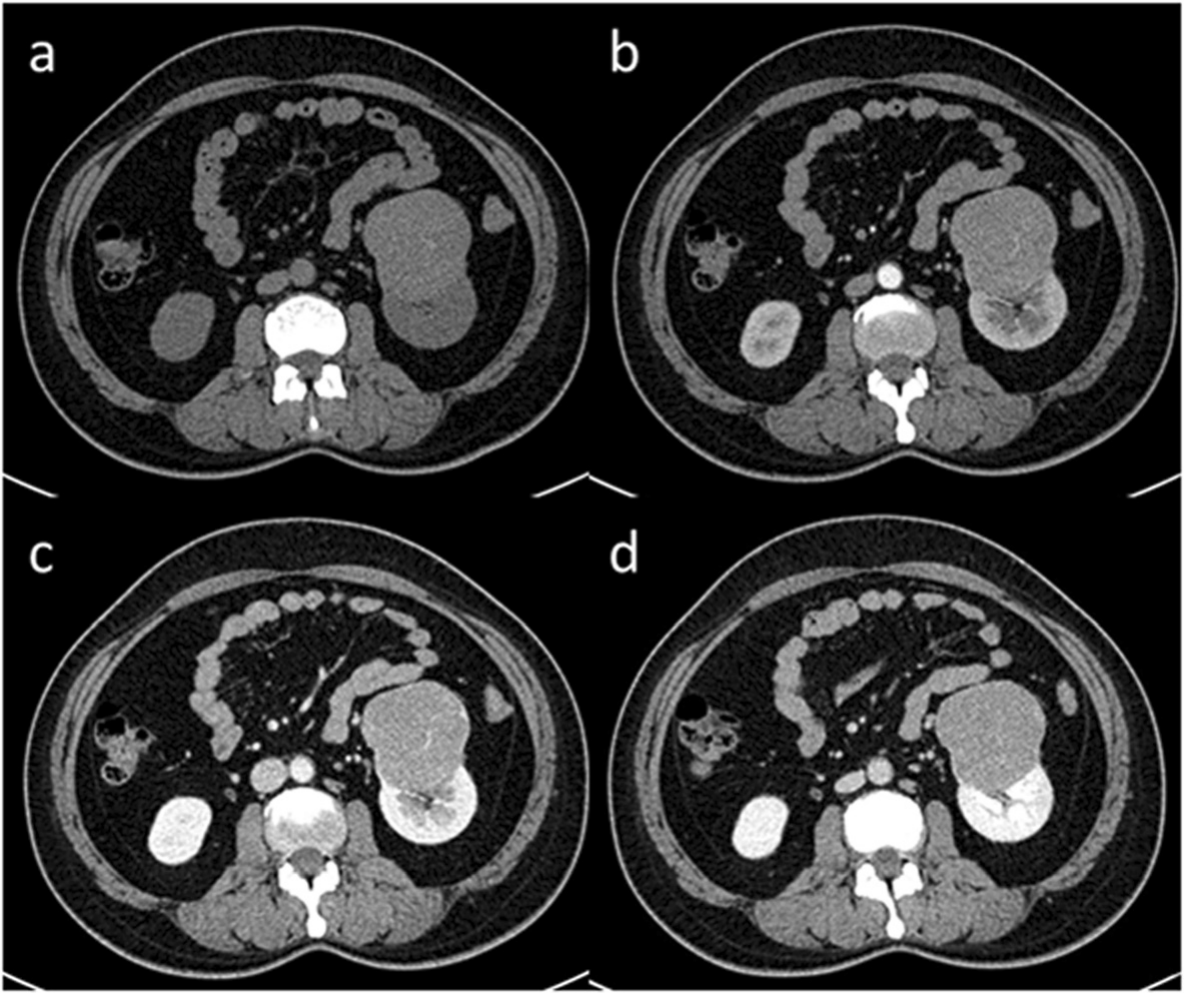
A 45-year-old woman with E-AML. The patient had no clinical symptoms. A renal mass was found during physical examination. Non-contrast axial CT image (a) demonstrates a hyper-density lesion with lobulated appearance in the lower renal pole of the left kidney. Contrast-enhanced CT images of arterial (b), venous (c), and delayed-phase (d) demonstrate relatively homogeneous enhancement
